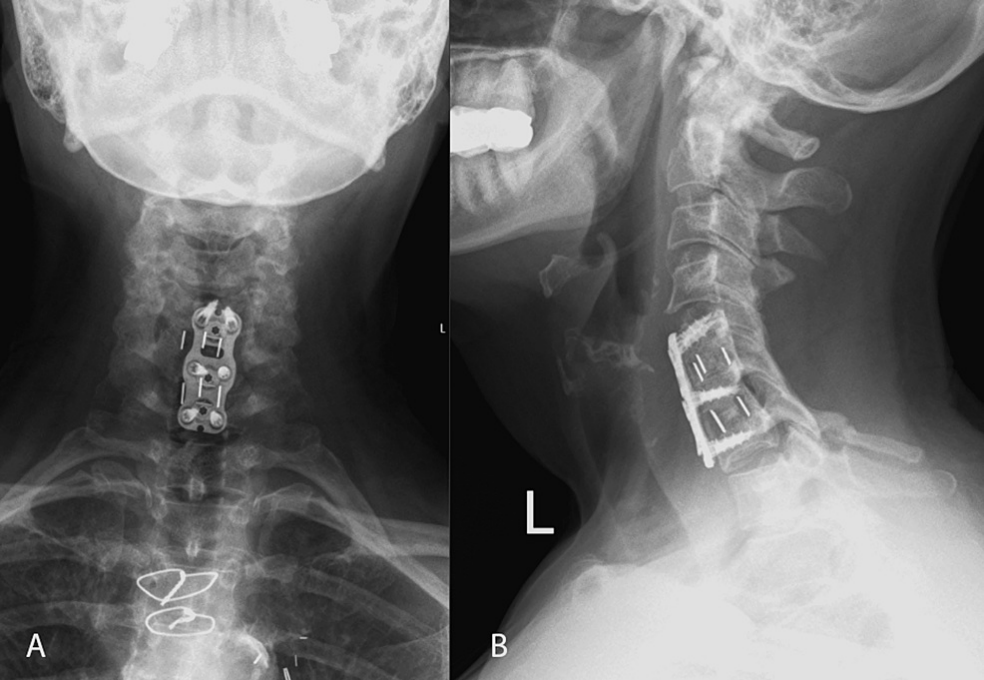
X-ray following C5-7 laminectomy and anterior cervical discectomy and fusion. An X-ray was taken 28 months post-operatively that demonstrated expected postsurgical changes with no complications. A: Coronal view; B: Sagittal view.
